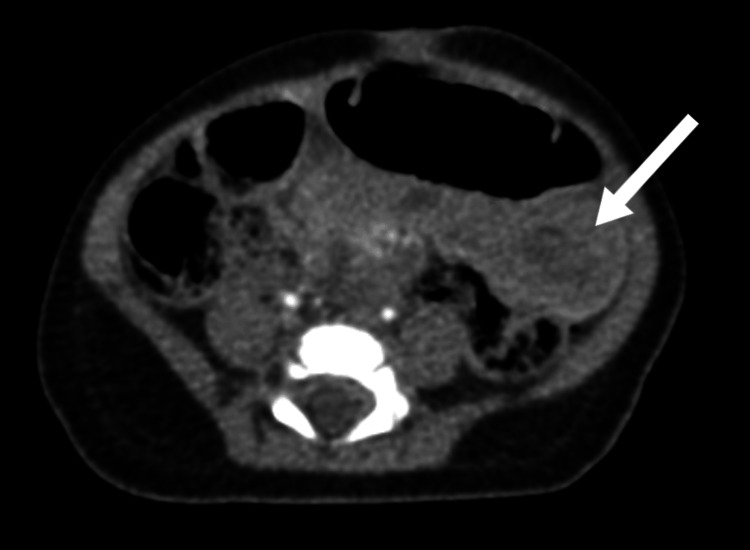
Axial CT of the mid-abdomen demonstrates ileo-ileal intussusception (white arrow). The walls of the bowel loops involved in the intussusception are thickened, showing average mural enhancement. No obvious lead point could be identified. It measures about 7.5 cm in length and 2.7 cm in short axis.
